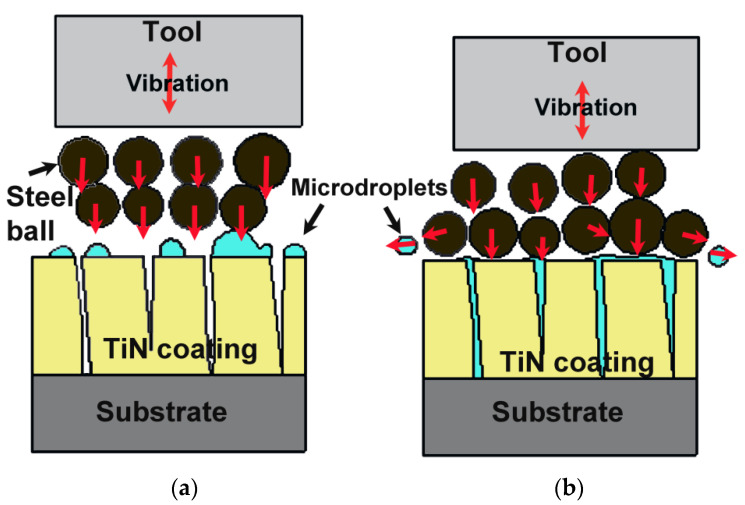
TiN-coated samples (a) before and (b) after being subjected to ultrasonic vibration.
